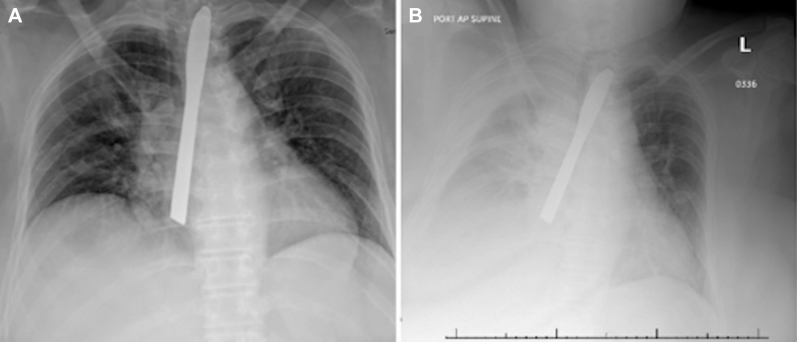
(A) Radiograph demonstrating a foreign body at the level of the esophagus. (B) Radiograph demonstrating a radiopaque knife overlying the midchest, presumed to be within the midesophagus, and interval development of a right-sided pleural effusion.
